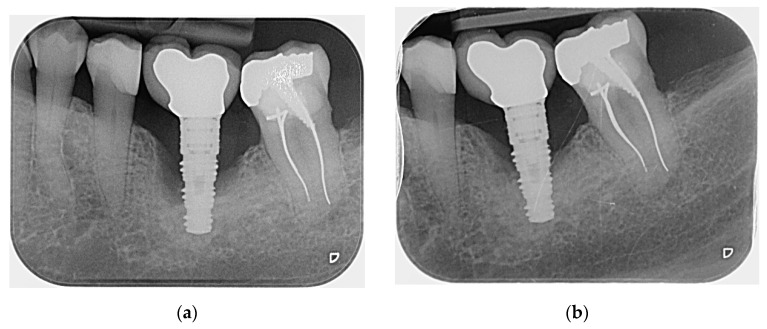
Radiographs of a peri-implant lesion before (a) and six months after (b) surgical treatment with the Ti-Brush^®^.
